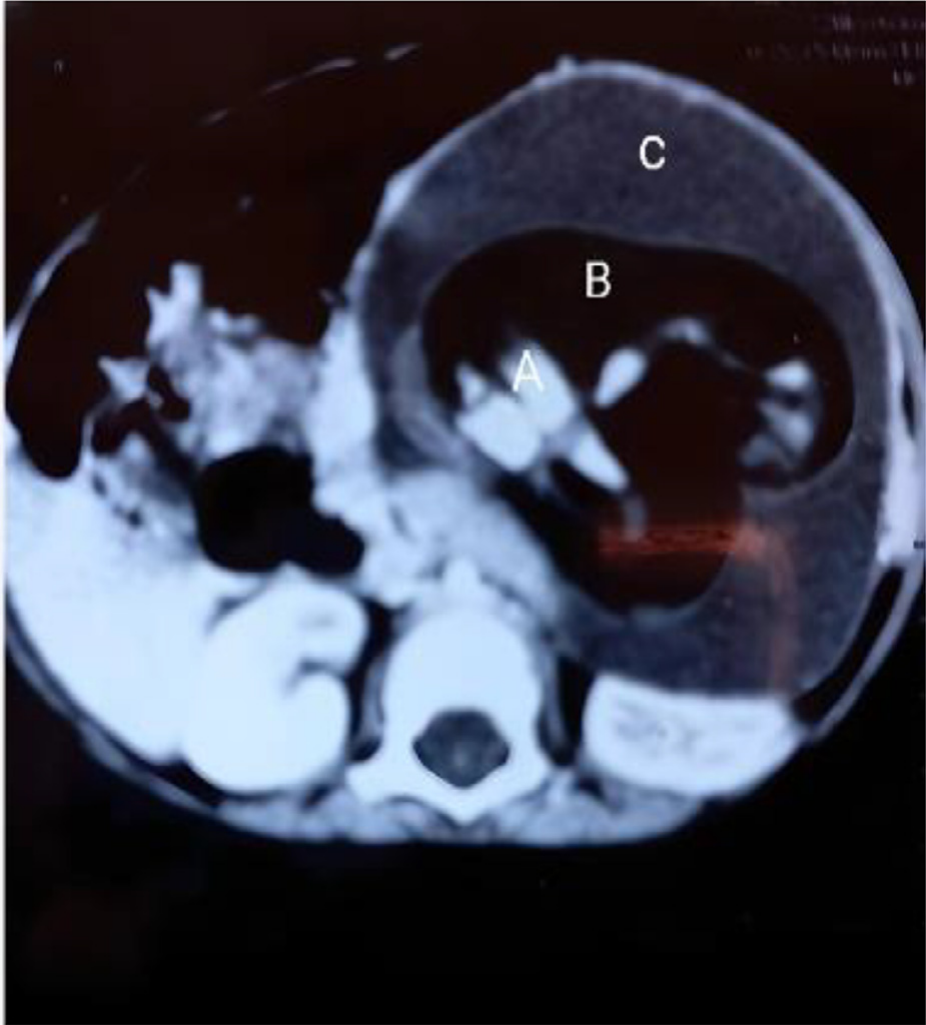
Axial view of CT scan showing a cystic structure in the abdomen (A: Soft tissue component, B: Gaseous component, C: Cystic fluid component).
